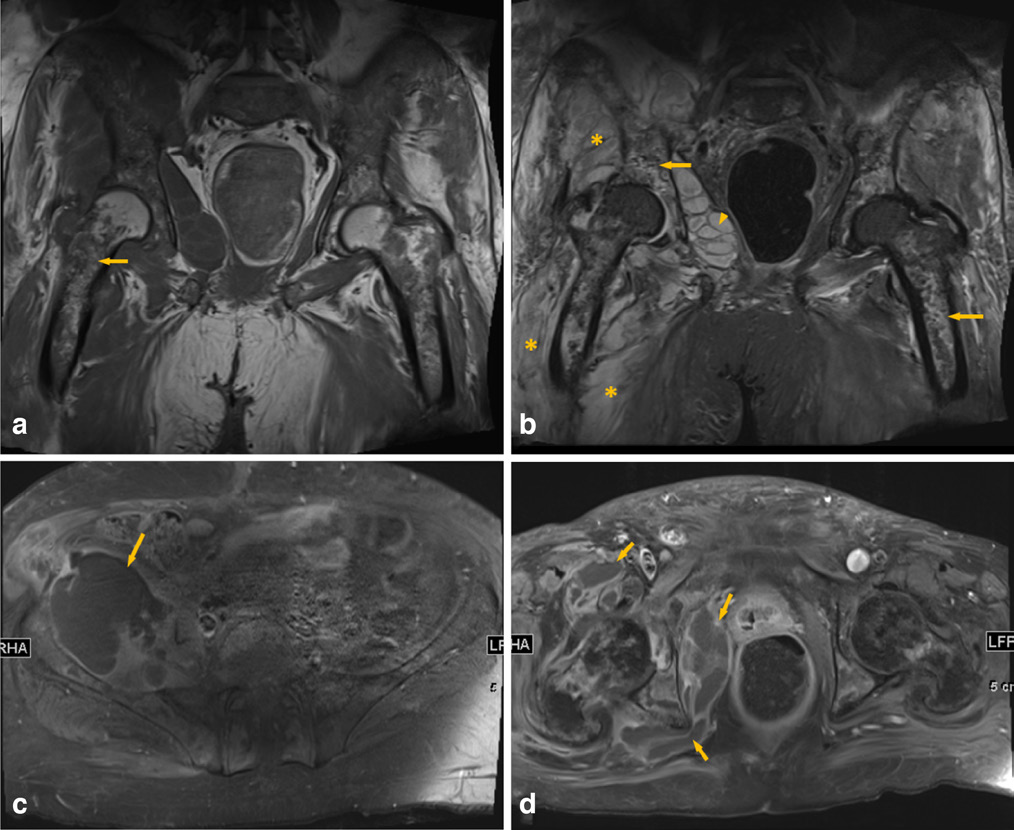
Around 1 month after initial presentation, coronal T1 (a) and fat saturated T2 (b) MRI of the pelvis show diffuse altered bone marrow signal intensity with areas of serpiginous low signal in T1 and high signal in T2 involving the pelvic and femoral bones, bilaterally indicating bone infarction/osteonecrosis (arrows). Associated multiple pelvic multiloculated fluid collections (arrowhead), as well as diffuse altered signal intensity of the pelvic muscles indicating myositis (asterisk) can be visualized as well. On axial post-contrast MRI (c, d), multiple pelvic collections are noted, the largest of which is in the right iliac crest muscle and around the left tuberosity (arrows).
